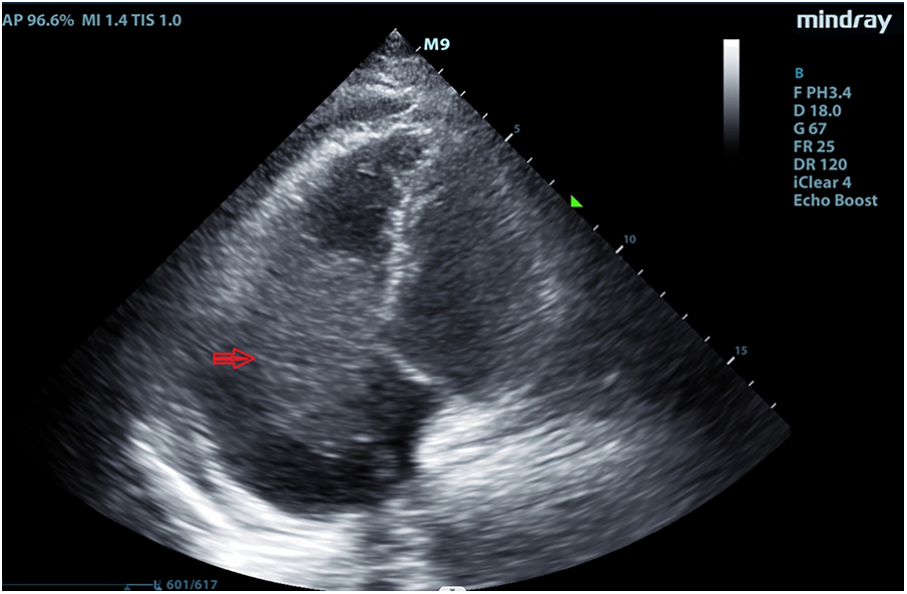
Echocardiography revealed a hypoechoic mass extending from the right atrium to the right ventricle in September 2, 2022.
